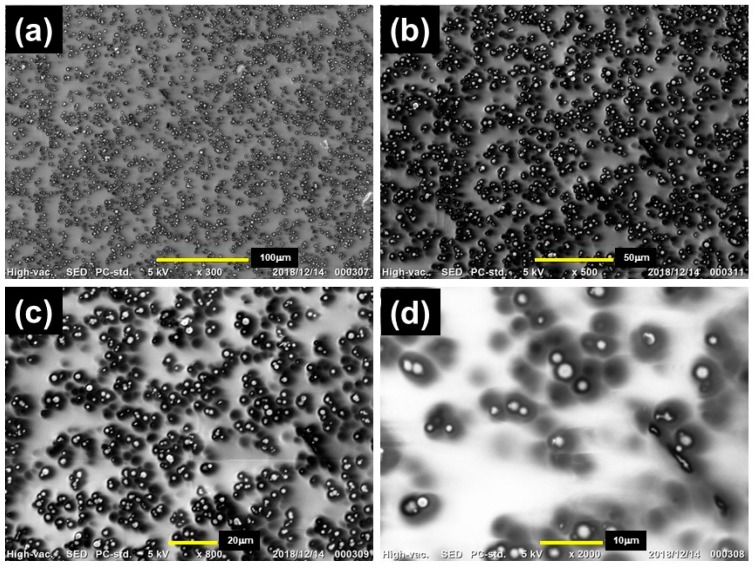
SEM photographs of a cross-section of the magnetic elastomer used in the CT observations at various magnifications; (a) ×300, (b) ×500, (c) ×800, (d) ×2000 (CI 70 wt%, DOP 70 wt%).
